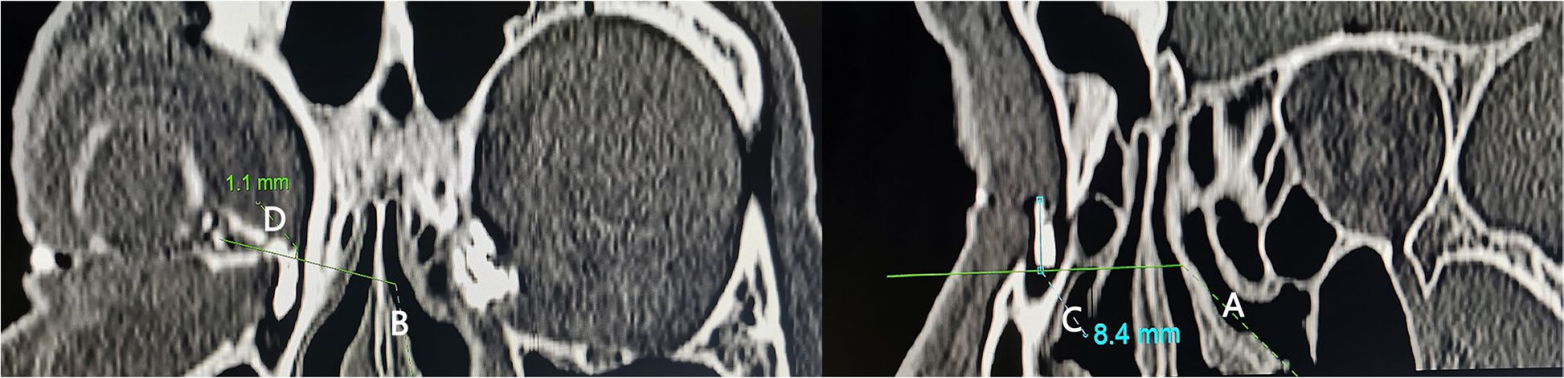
Measurements in the long axis of a small lacrimal sac on computed tomographic dacryocystography (CT-DCG) parasagittal images. For most patients, it is difficult to display the common canaliculus (CC) and the middle turbinate axilla (MTA) simultaneously on coronal CT-DCG images, and it is difficult to measure their distance directly. In this study, the distance from the MTA to the CC was calculated by the difference between the height from the SSF to the MTA and the height of the sac above the CC. A, a line drew from the middle turbinate axilla (MTA) to the long axis of the sac at right angles. B, a line drawn through the common canaliculus (CC) to the long axis of the sac at right angles. C, the height of the sac above the MTA. D, the height of the sac above the CC
